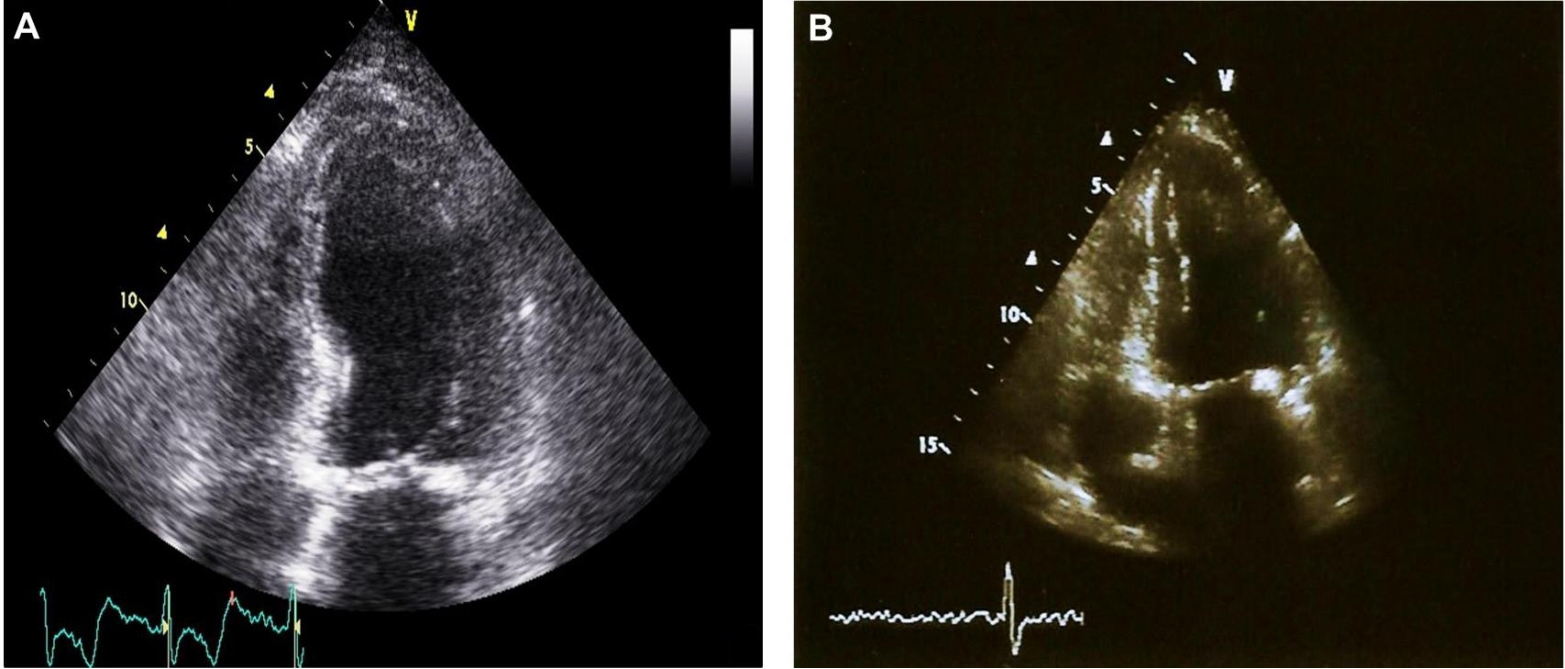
Echocardiogram on second presentation and follow-up. (A) Echocardiogram revealing akinesis of the apex during the patient's second presentation to our hospital. (B) Echocardiogram showing normally contracting apex with complete recovery of ejection fraction after the patient's second presentation.
